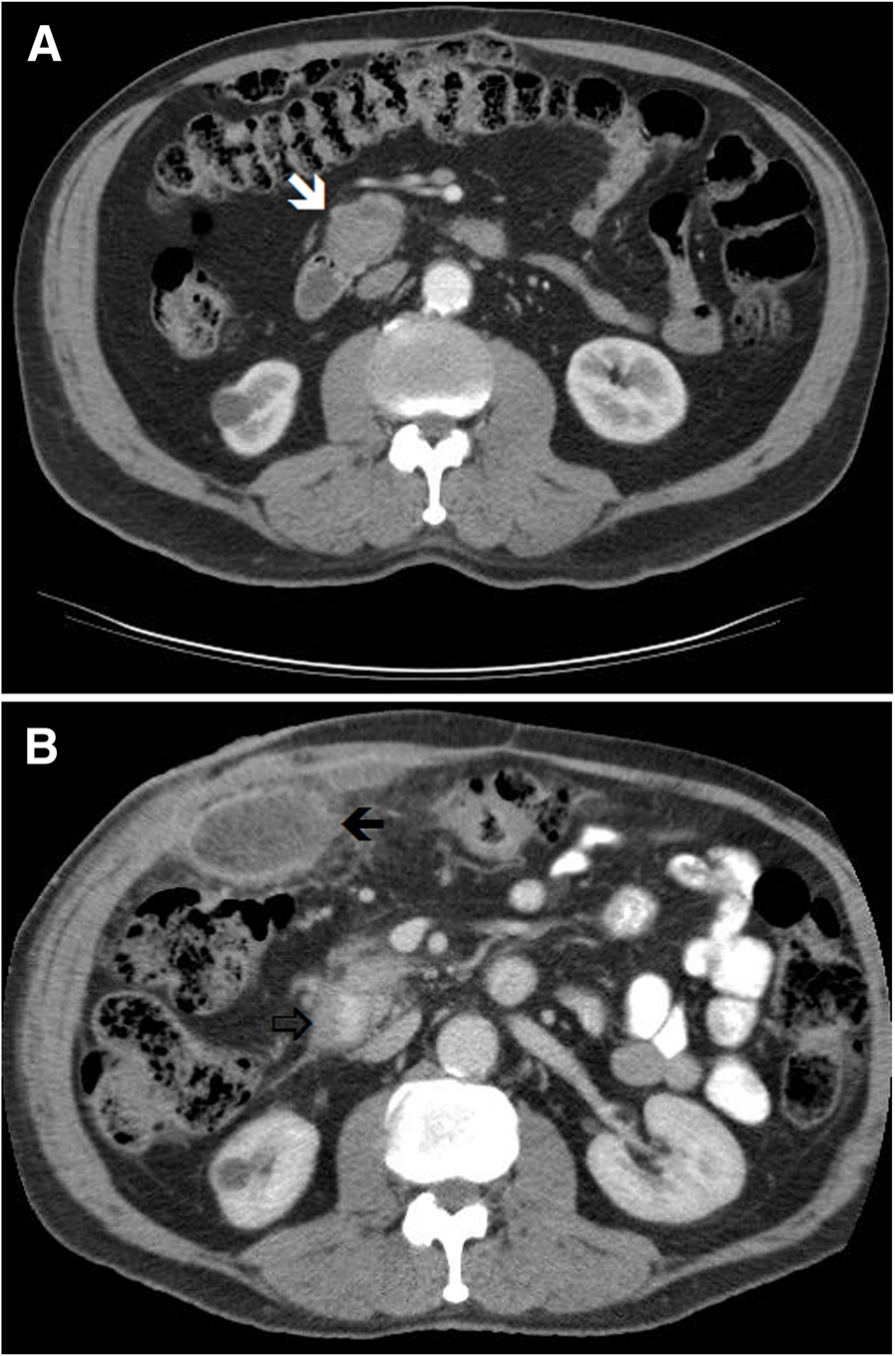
CT images of the first patient. (A) An 3 cm lesion was seen on initial post contrast venous phase CT (white arrow). This was mis-interpreted as a pancreatic malignancy. (B) Patient underwent a laparotomy which failed to identify a mass in the pancreas. Post operatively, he developed a wound site abscess (black arrow).
